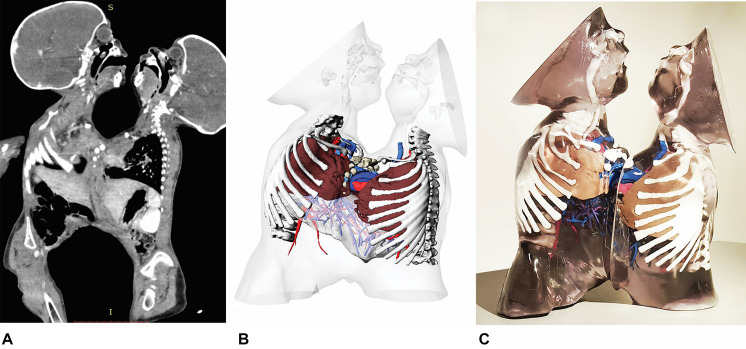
CT sagittal images of the entire conjoined anatomical structures (A), 3D virtual reconstruction of patient’s anatomy (B), multi-color and multi-material 3D printed anatomical model (C).
